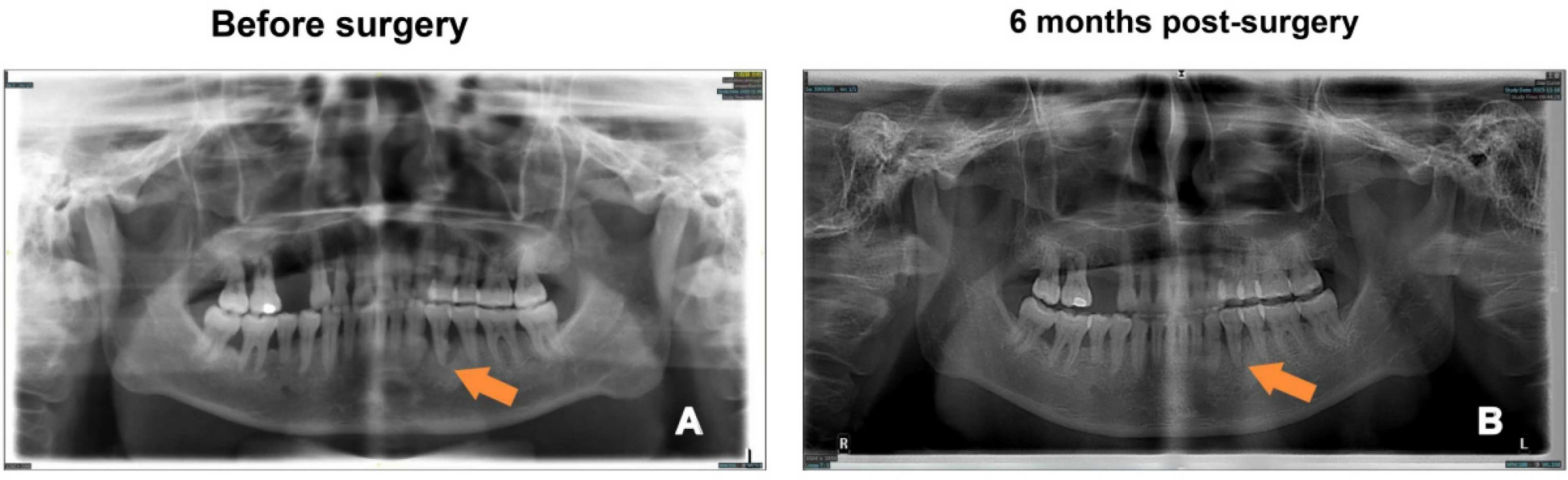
Radiographic comparison of periodontal health before and after surgery. (A) Preoperative radiograph showing the periodontal condition before surgery (arrow indicates the area of concern); (B) Radiograph taken 6 months post-surgery, showing improvement in periodontal health (arrow indicates the healed area).
